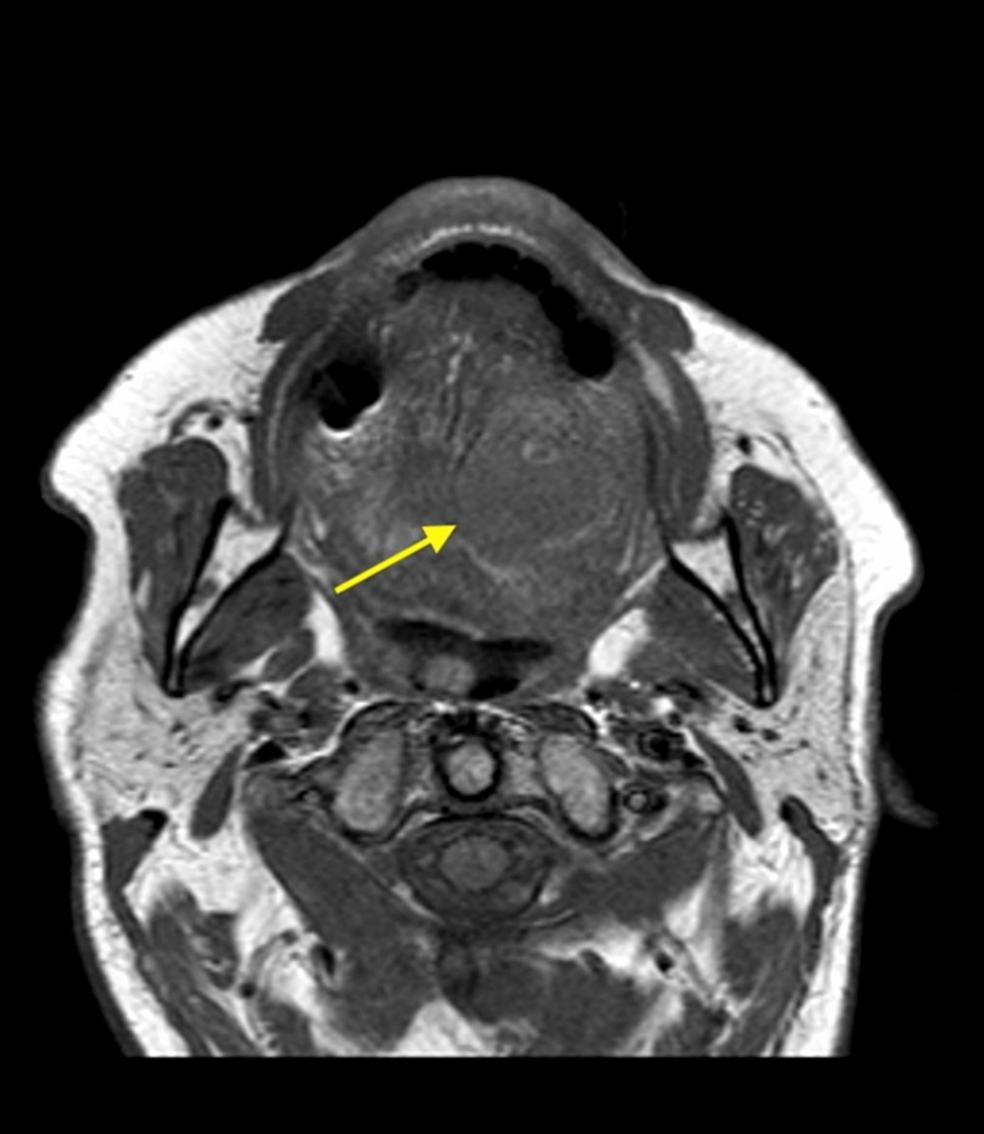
Axial T1-weighted pre-contrast magnetic resonance image of the neck showing an isointense 2.7 cm ovoid lesion within the tongue (arrow).
